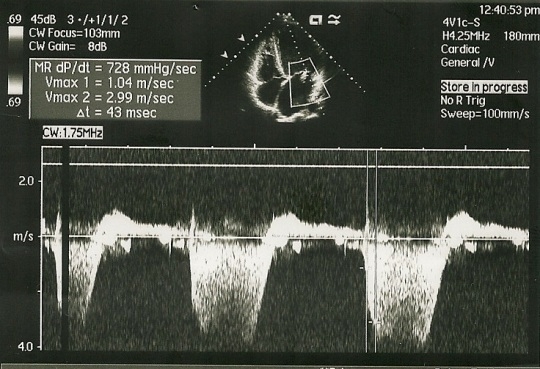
LV filling pattern at echocardiography
